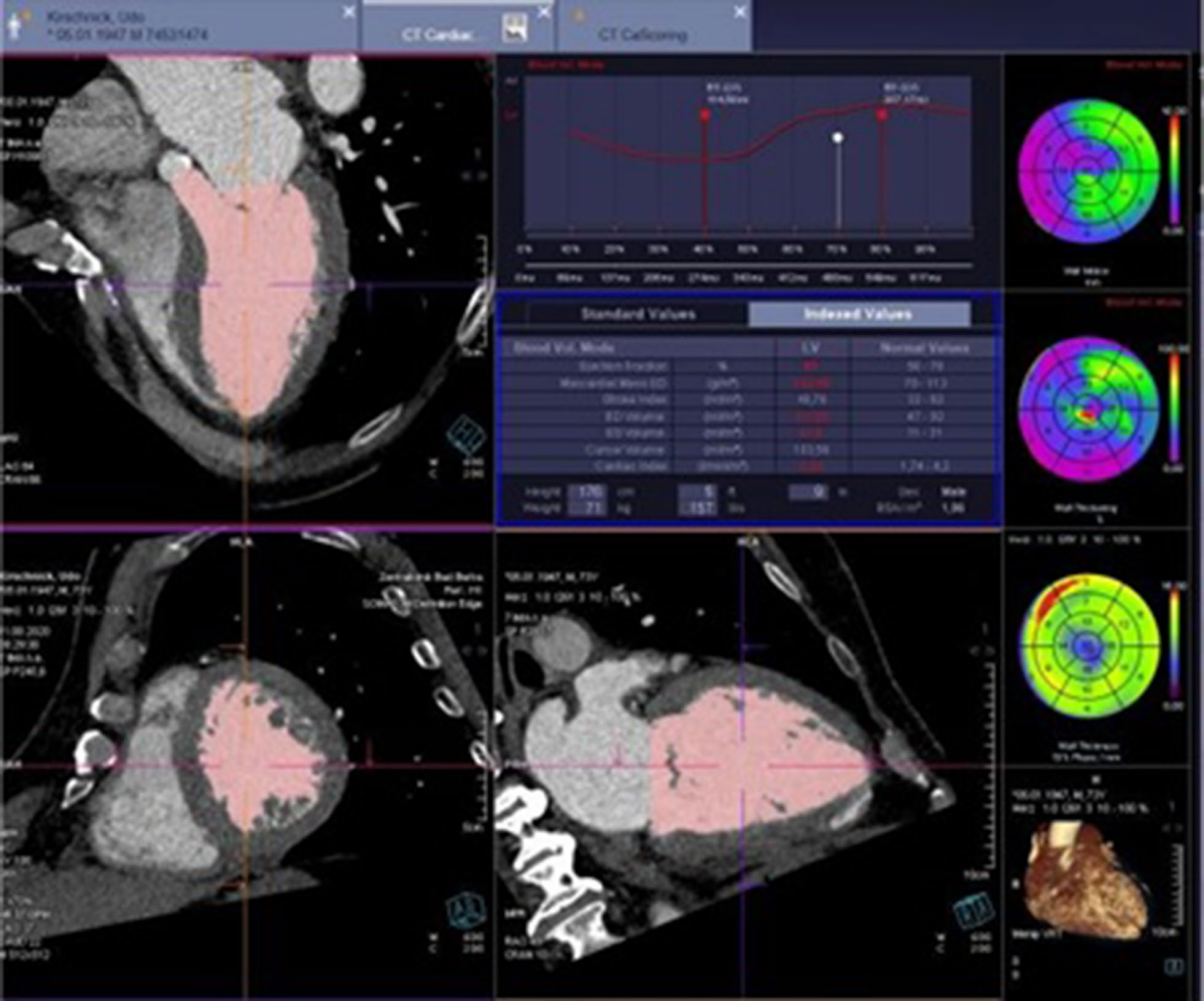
The use of the CT to measure the stroke volume especially in LG aortic stenosis
